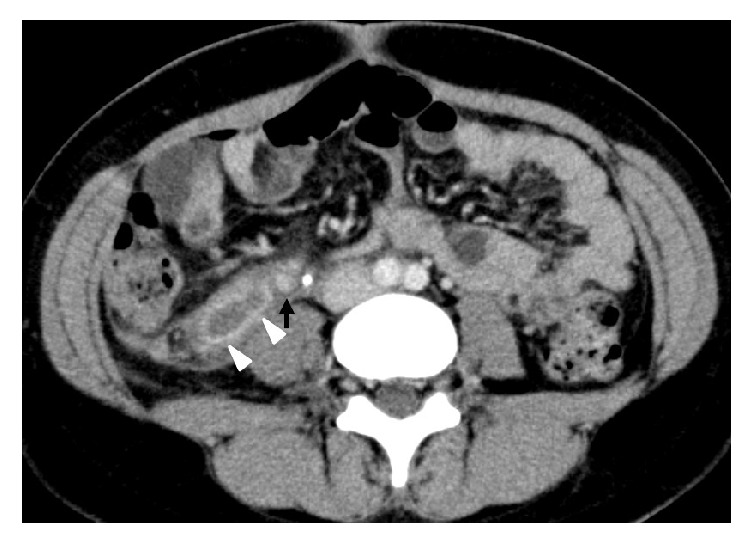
Abdominal dynamic CT scan (delayed phase). A ring-enhanced right retroperitoneal mass (white arrowhead). It was distinct from the right ovarian vein (black arrow).
